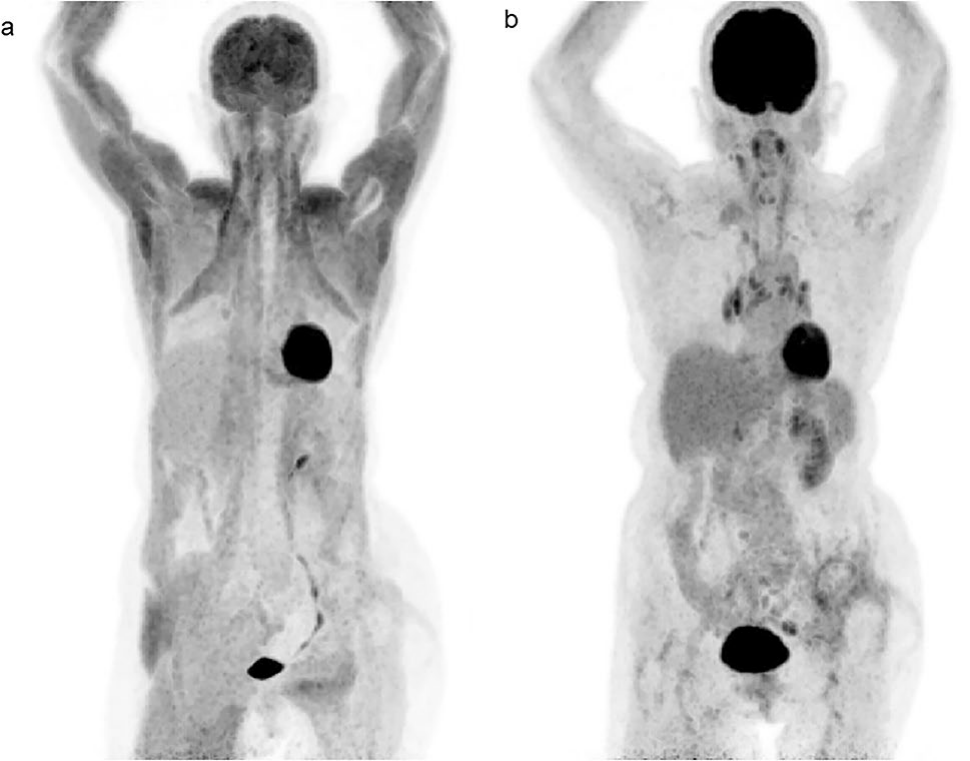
a A patient who had eaten a meal within 4 h from injection of FDG. b Restudy of FDG-PET/CT after one month in the same patient. Abnormal uptake in whole-body muscle and decreased FDG uptake in the brain were seen in (a), despite a BS level of 120 mg/dL at the time of FDG injection, has disappeared in the restudy (b). BS levels typically decrease to normal levels approximately two hours after the last food intake except in patients with diabetes. For this reason, it is necessary to conduct a detailed interview with the patient before the FDG injection, in addition to checking their BS level
